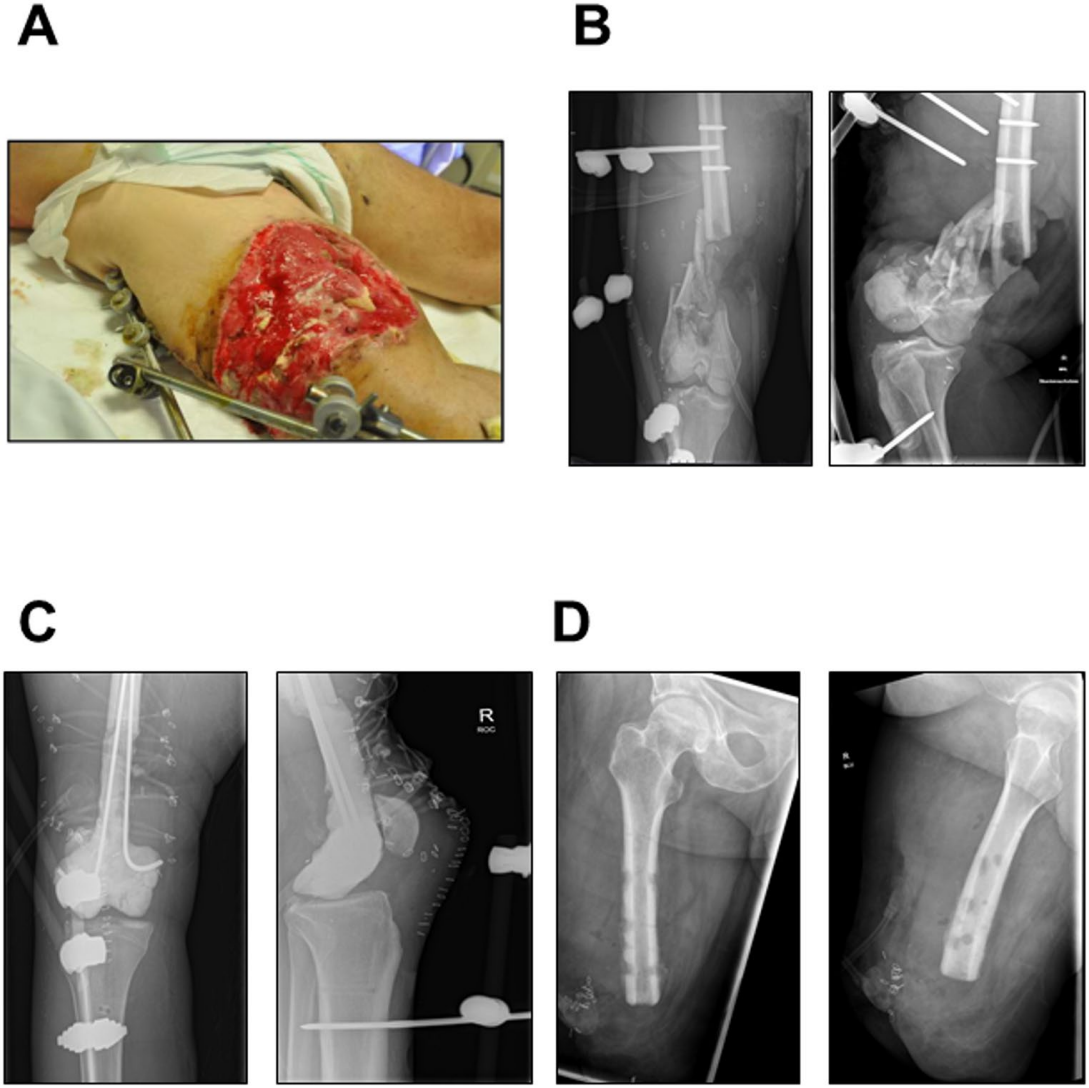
Fig. 1 (A) Clinical wound situation of the right lower extremity on the day of admission to our hospital. (B) Preoperative a.p. (anterior-posterior) (left) and lateral (right) x-ray of the right knee on the day of admission to our hospital. (C) Postoperative a.p. (left) and lateral (right) x-ray of the right knee following treatment with a PALACOS^®^ cement spacer and re-osteosynthesis using an external fixator and wire osteosynthesis. (D) Postoperative a.p. (left) and lateral (right) x-ray after major amputation of the right lower extremity. All images from our own hospital
